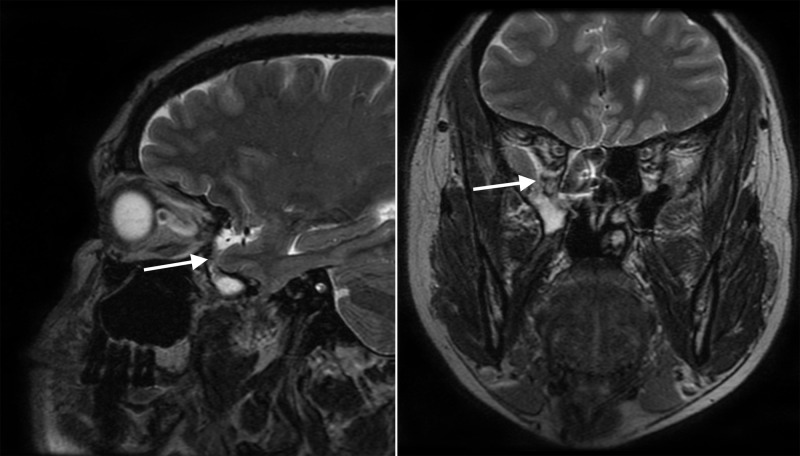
MRI of the brain without contrast Magnetic resonance imaging (MRI) of the brain demonstrates an empty sella, intracranial hypertension, and a right middle fossa encephalocele associated with a possible CSF fistula.
